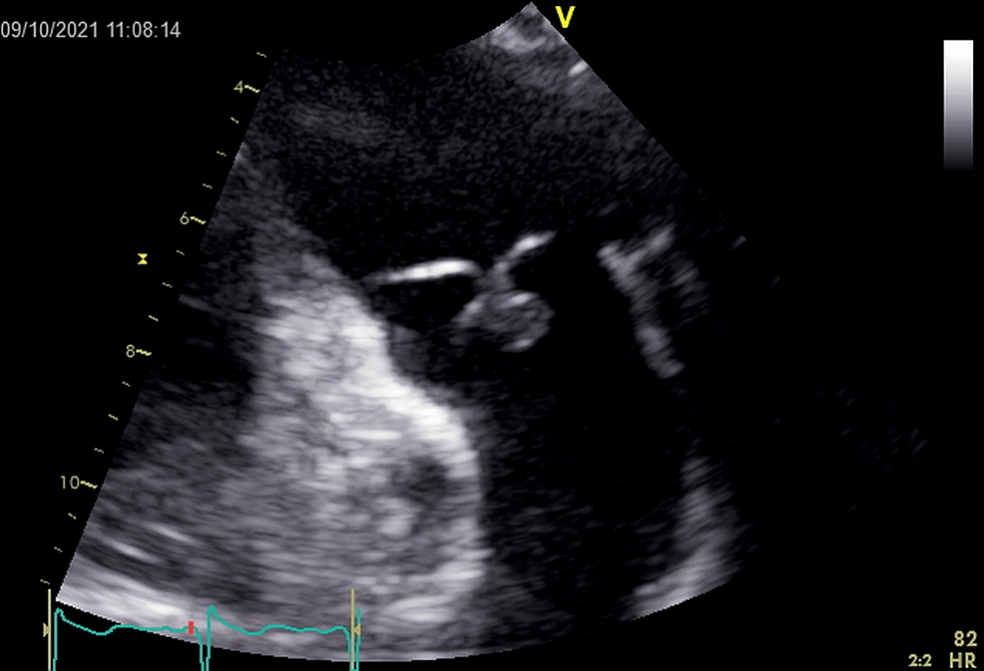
Transthoracic echocardiogram showing 0.7 X 0.9 cm mobile structure seen on the pulmonic valve leaflet
